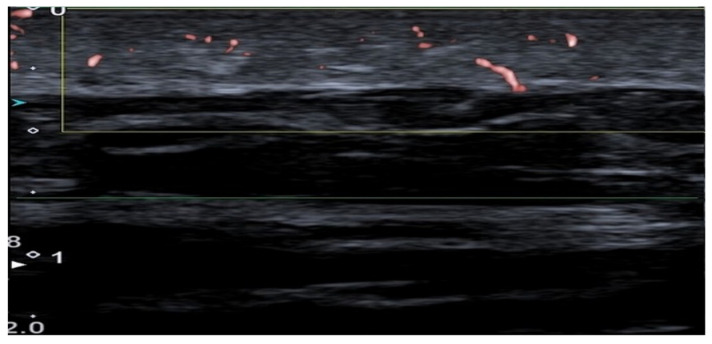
Superb Microvascular Imaging (SMI) of the dermal vascularization in the forearm (upper part of the image), with 1933 colored pixels, and with Power Doppler (lower part of the image), with 0 colored pixels. This image was published under open access, CC-BY 4.0 rights, and originates from [10].
